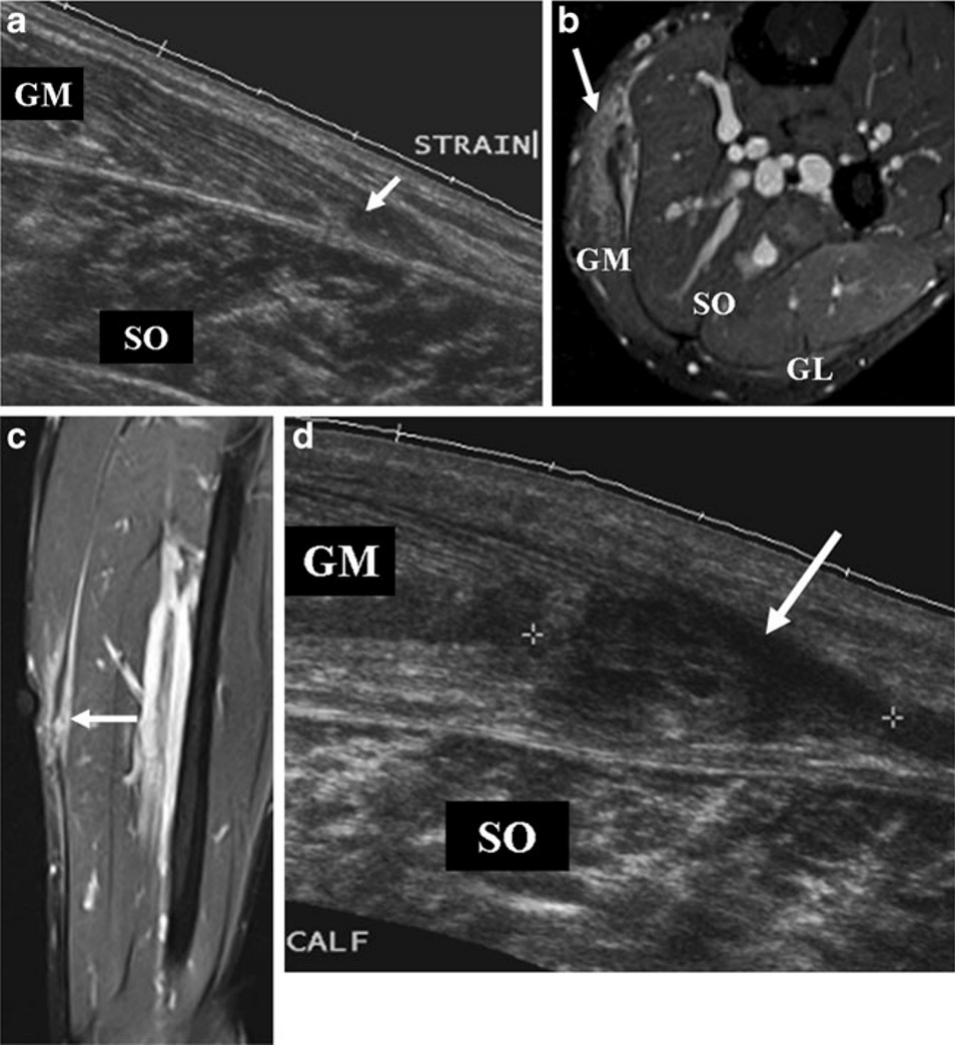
A 27-year-old football player presenting with left calf pain, clinically diagnosed as grade 2 injury. a On ultrasound at initial presentation, a hypoechoic area measuring 1.0 × 0.4 cm was noted (*arrow*), corresponding with a partial tear of the medial head of the gastrocnemius (*GM*). Colour Doppler imaging was normal (not shown). No evidence of haematoma or other abnormality was observed. Soleus muscle (*SO*) appears intact. MR imaging was not performed on this patient at this time. b-d Follow-up imaging 2 months after initial presentation. b Axial and (c) coronal FS T2-w TSE images reveal areas of hyperintensity with feathery appearance, consistent with a partial tear of the medial head of the gastrocnemius muscle with intramuscular oedema (*arrow*). Note the peritendinous oedema. d On ultrasound, a persistent hypoechogenic area was noted that appeared to have increased in extent (*arrow*). Colour Doppler imaging was normal (not shown). A 7-month follow-up MR imaging showed complete recovery (not shown)
